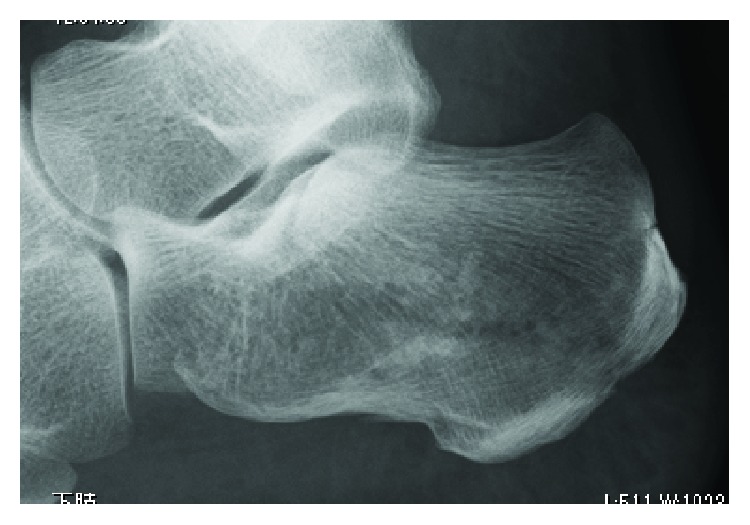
Lateral radiograph of the left foot following injury. X-ray shows the tongue-type fracture of the left calcaneus.
